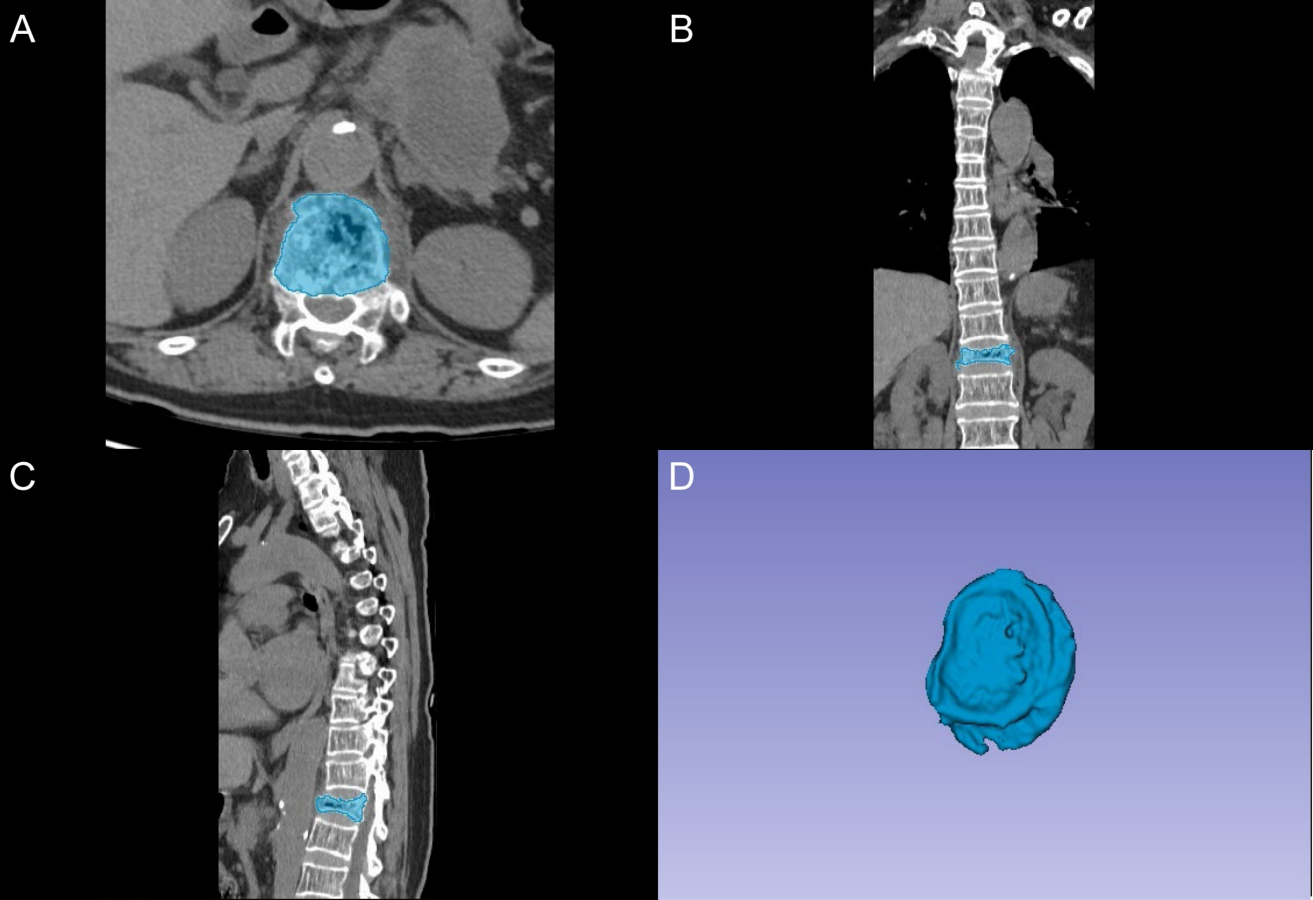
Vertebral segmentation protocol and three-dimensional reconstruction. A: Axial CT image showing semi-automatic segmentation of the vertebral body (blue). B: Coronal reconstruction demonstrating the segmented vertebral region. C: Sagittal view of the segmented vertebral body. D: Three-dimensional reconstruction of the segmented vertebral volume of interest.
